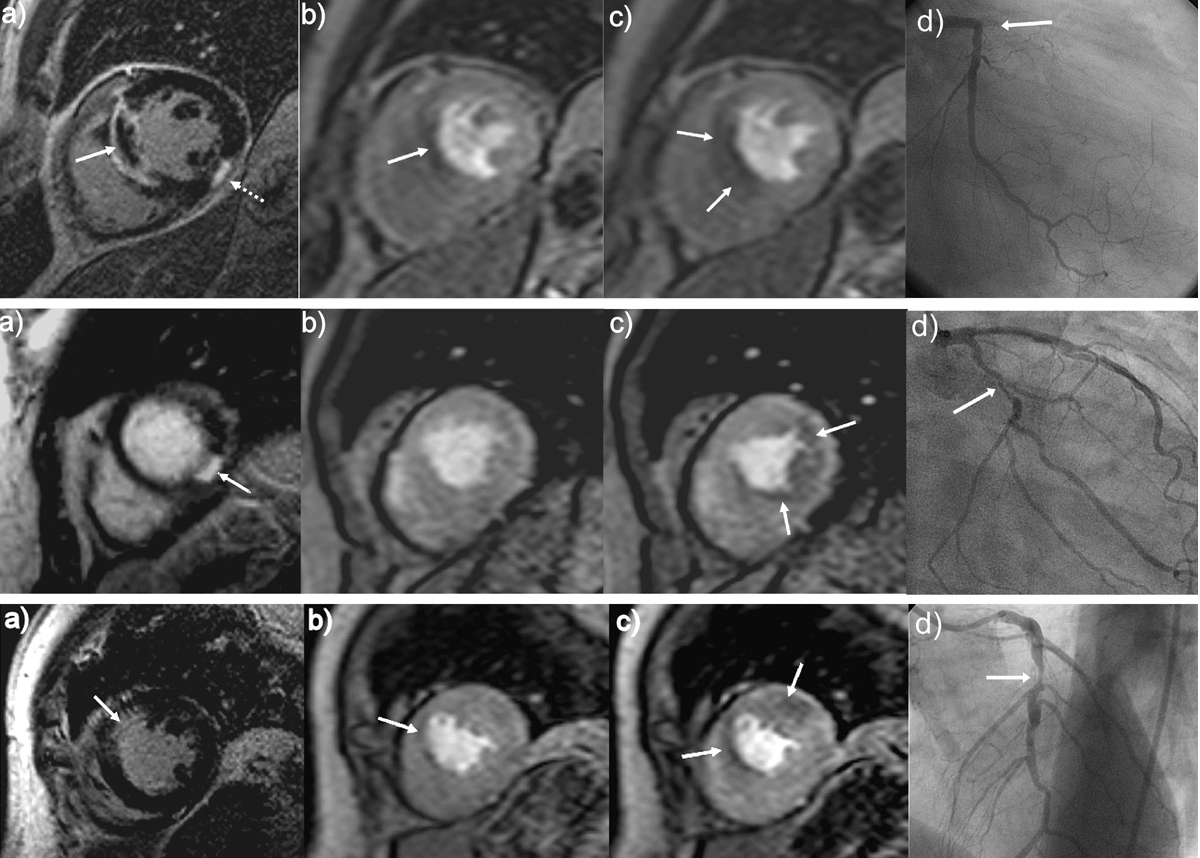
Column A *= Late gadolinium-enhancement CMR in the short axis.*Column B *= Rest perfusion CMR at peak myocardial enhancement in the identical location. *Column C *= Adenosine stress perfusion CMR with identical image parameters to column B. *Column D *= Corresponding X-ray coronary angiogram. *Top Row *= Example of Q-wave STEMI, showing a large septal scar with a central area of microvascular obstruction (A; full arrow). There is also a small inferior scar (A; dotted arrow). Rest perfusion CMR (B) shows a defect corresponding predominantly to the area of the microvascular obstruction. Stress perfusion CMR (C) shows the perfusion defect in the entire infarct and extending marginally into the peri-infarct zone. Coronary angiography (D) revealed an occluded proximal LAD at the site of a previous stent. *Middle Row *= Example of Non Q-wave STEMI, showing a small inferior scar (A; arrow). Rest perfusion CMR (B) shows the small inferior scar is not detected as a fixed perfusion defect. The stress perfusion image (C) shows a large inducible perfusion defect infero-laterally, extending beyond the scar into the peri-infarct zone (C; arrow). The coronary angiogram (D) shows a severe stenosis in the mid circumflex artery. *Bottom Row *= Example of NSTEMI, showing a small subendocardial scar in the antero-septal segment (A). Rest perfusion CMR (B) appears homogenous outside the scar. The stress perfusion image (C) shows a large area of inducible antero-septal ischemia. On coronary angiography (D), severe disease in the left anterior descending artery was found.
